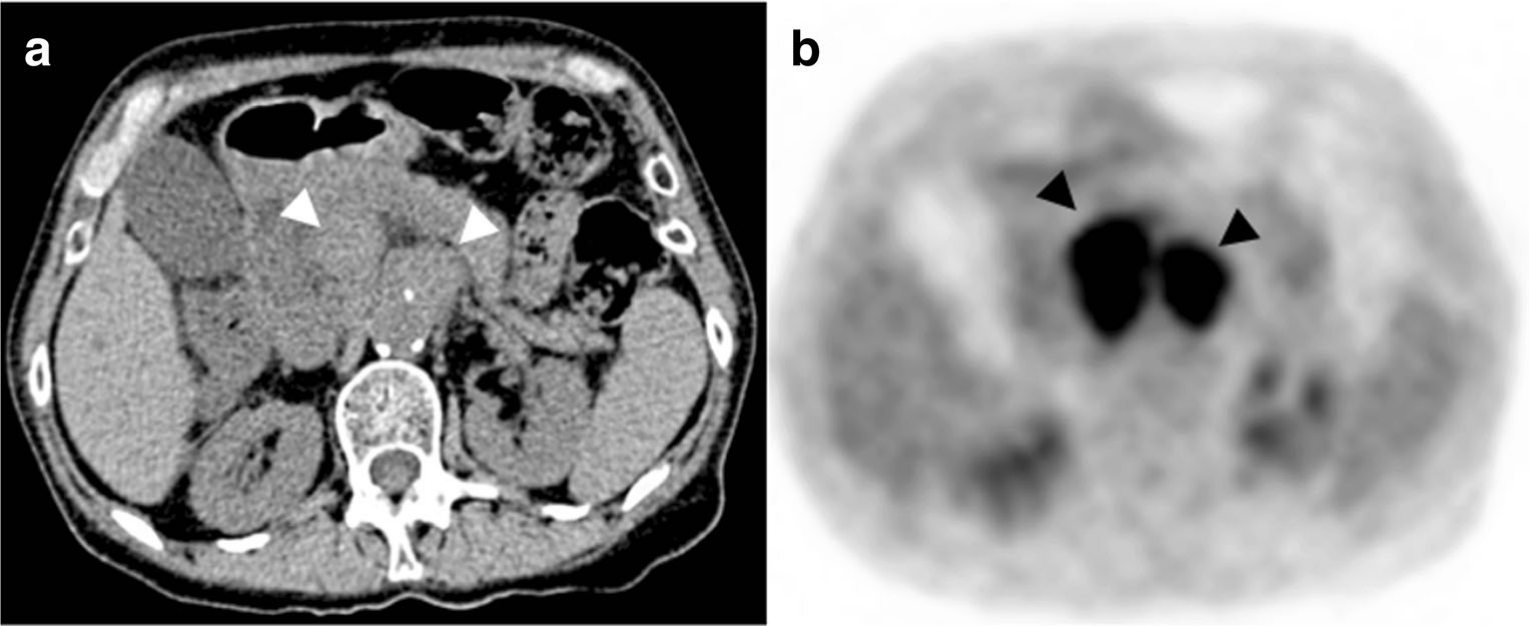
Post-transplantation lymphoproliferative disease (PTLD). Weight loss and general alteration. Voluminous retroperitoneal lymphadenopathies on non-enhanced abdominal CT (*arrowheads*) (a) with increased metabolism on FDG-PET CT scanning (*arrowheads*) (b)
